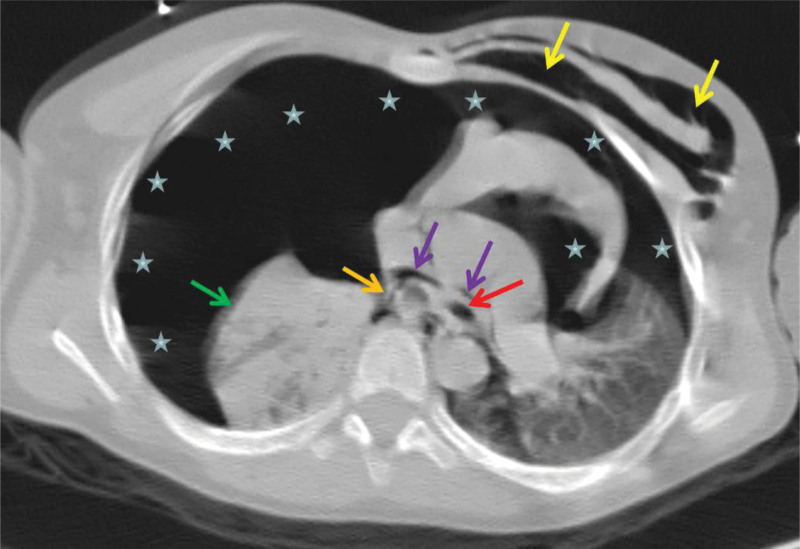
The image of CT scan. The left main bronchus demonstrated adequate gas filling and intact wall integrity (as indicated by the red arrow). Conversely, the right main bronchus exhibited collapse with poor wall continuity (as shown by the orange arrow). There was associated right lung collapse and atelectasis (as highlighted by the green arrow). A substantial pneumothorax was observed on the right side, extending across the mediastinum anteriorly into the left chest cavity (as depicted by the stars). Mediastinal air accumulation was evident (as denoted by the purple arrow), collectively suggesting a rupture in the right bronchus. Notably, there was air entrapment along the left chest wall in this image (indicated by the yellow arrow). CT = computed tomography.
